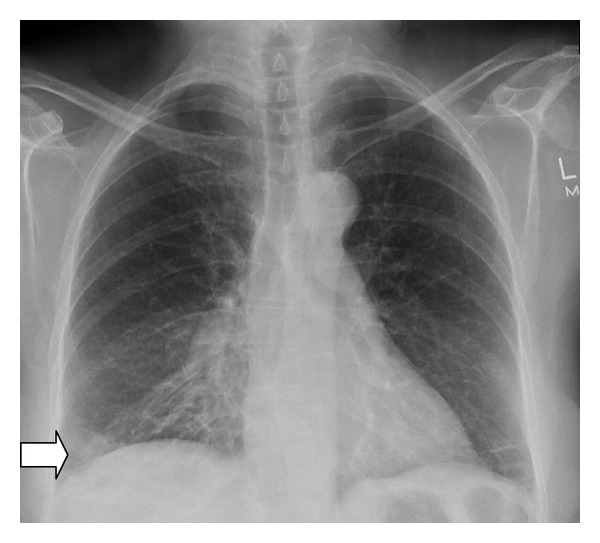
PA chest X-ray showing ill-defined opacity right lower zone (arrow).
